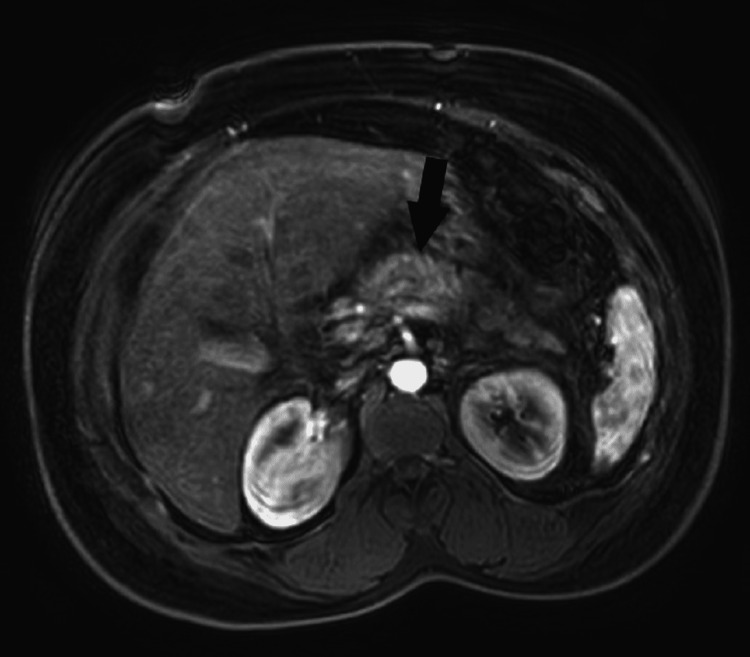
MRI abdomen with MRCP showing irregular contour of pancreas and swelling. MRCP: magnetic resonance imaging with cholangiopancreatography
